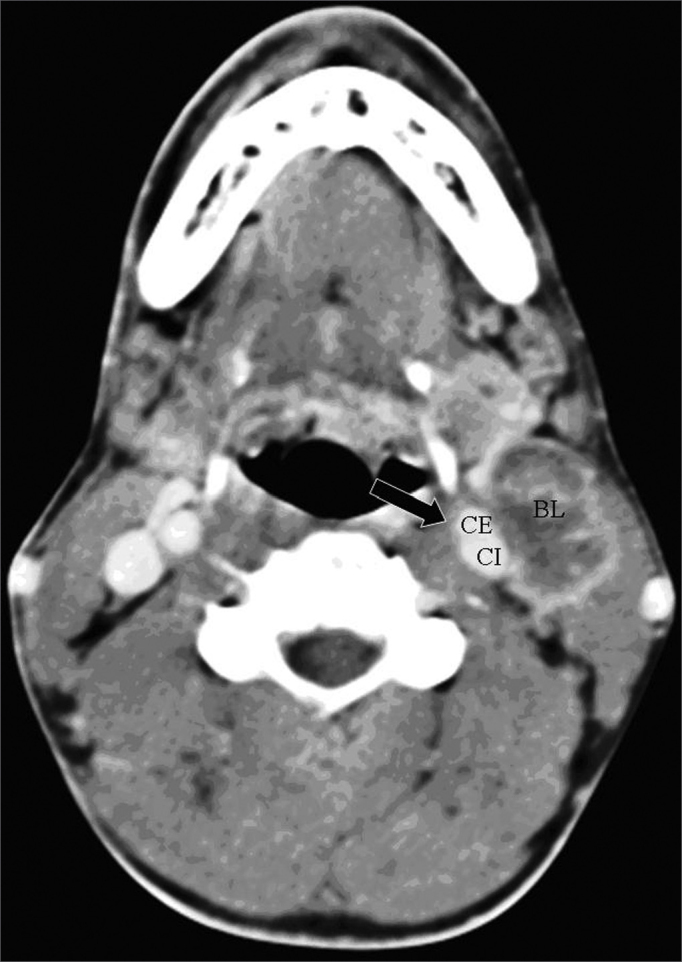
axial CT (4 sequential sections) with endovenous contrast. High jugular-carotid (level II) lymph node block (BL) to the left with signs of rupture of the capsule and a contact interface over 50% of the internal carotid artery circumference (arrow).
